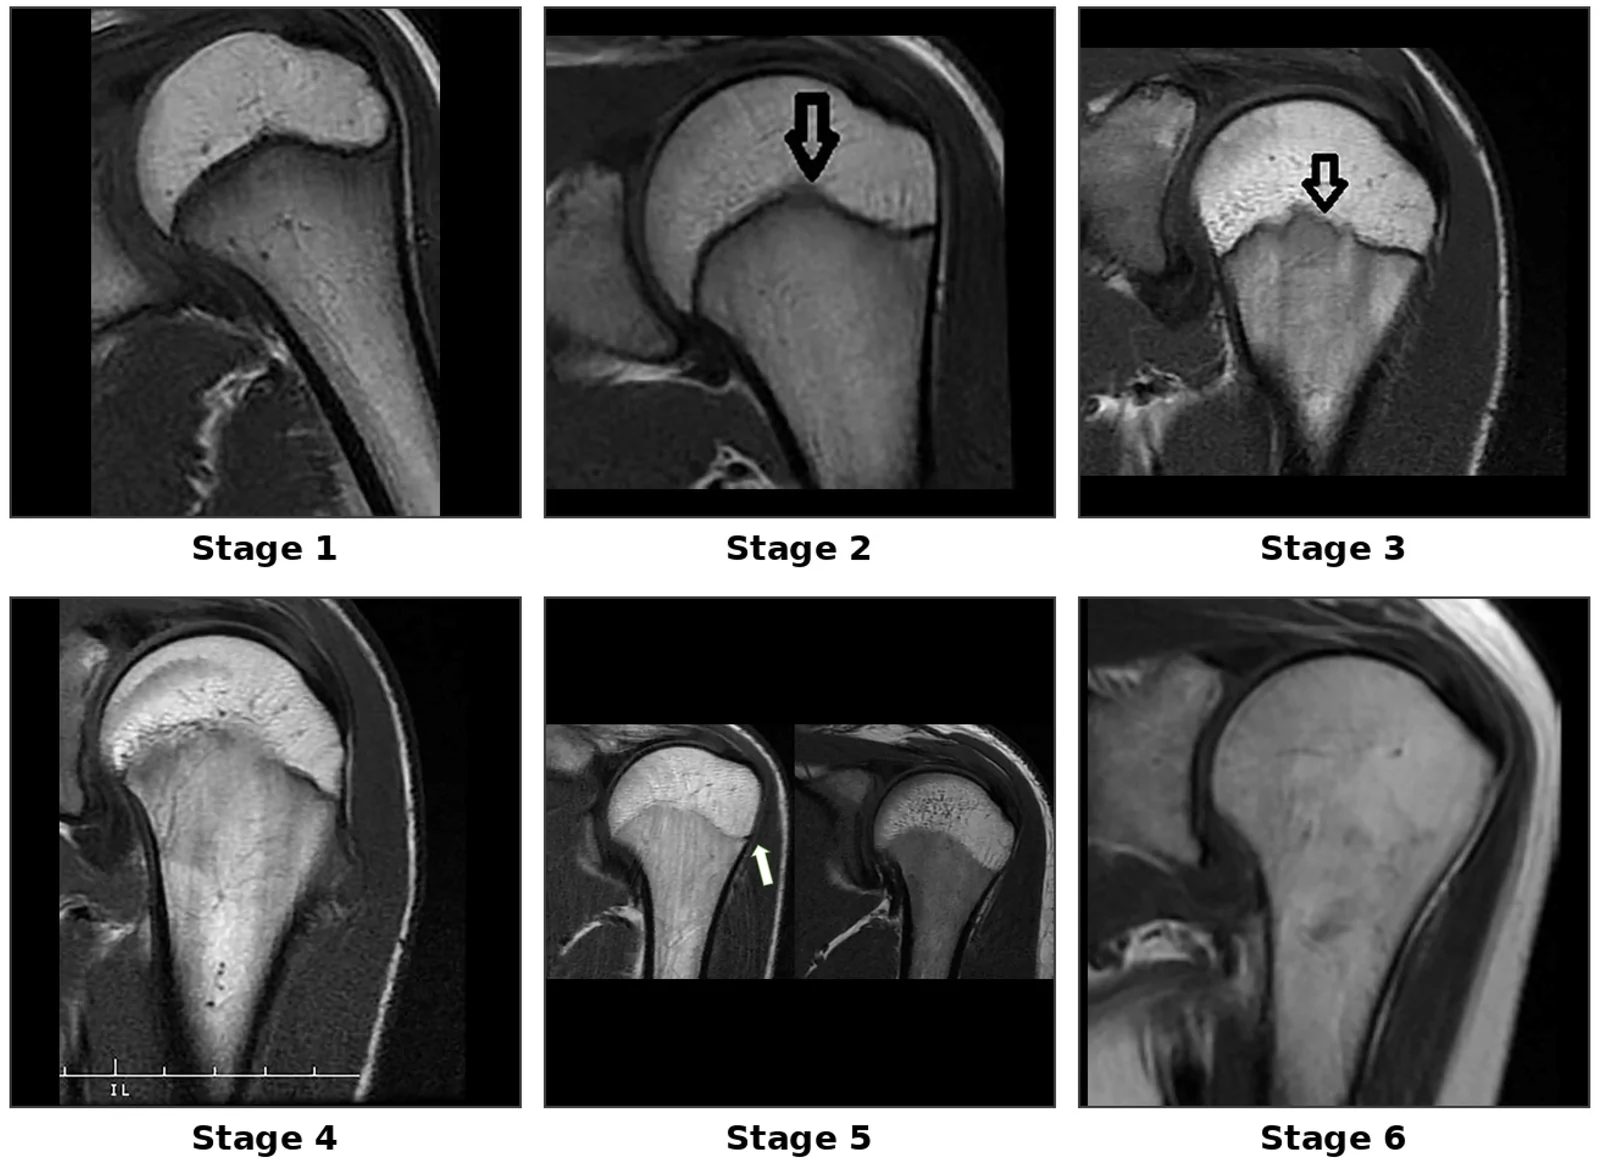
Six ossification stages of the proximal humeral epiphysis on coronal oblique T1-weighted MRI.
